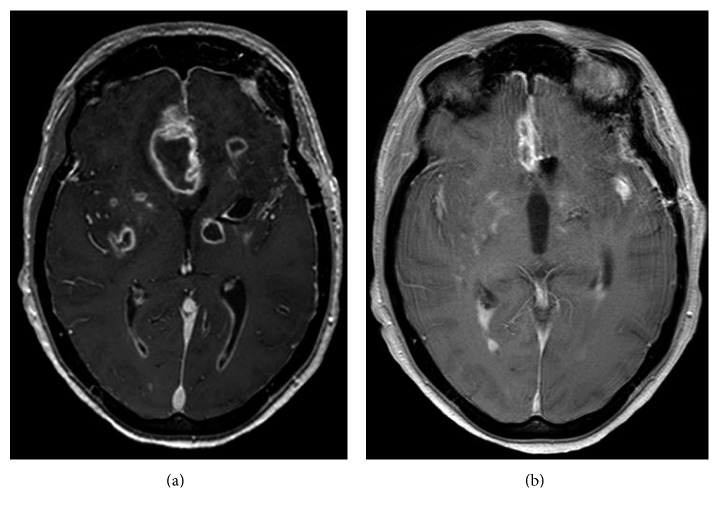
Brain MRI. Contrast-enhanced axial transverse T1-weighted view showing multiple necrotic and cystic lesions appearing as low signal intensity areas surrounded by an intensely enhanced rim corresponding to the pyogenic membrane (a). There was a regression of the lesions three weeks later (b).
